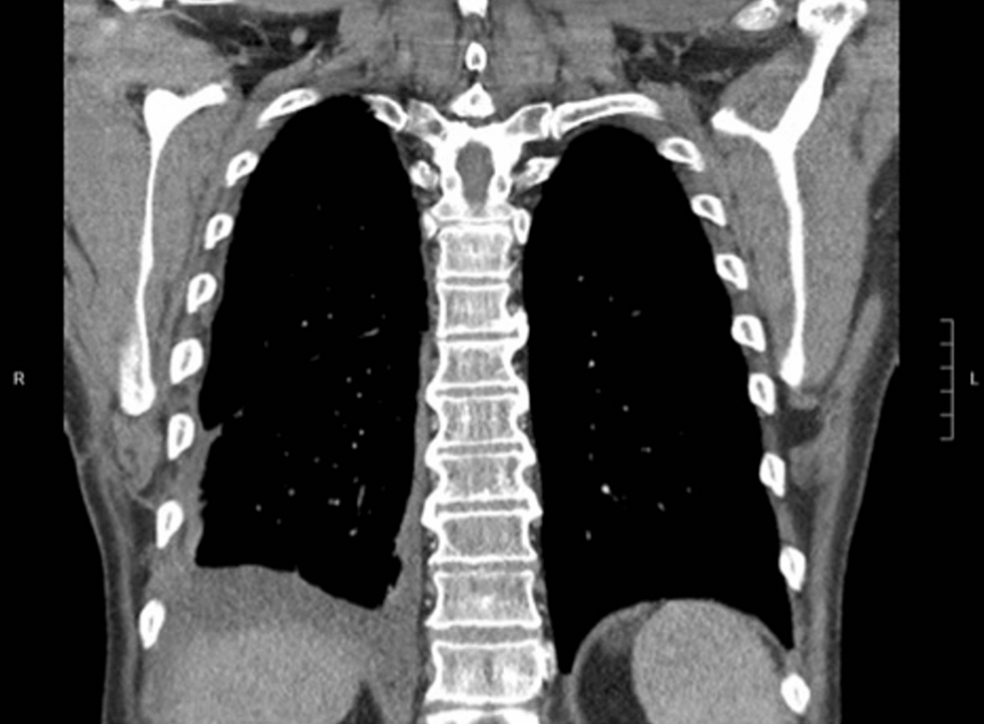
Chest CT with intravenous contrast at nine weeks after initial discharge, eight weeks after treatment. Significantly less pronounced pleural masses with small right-sided pleural effusion.
